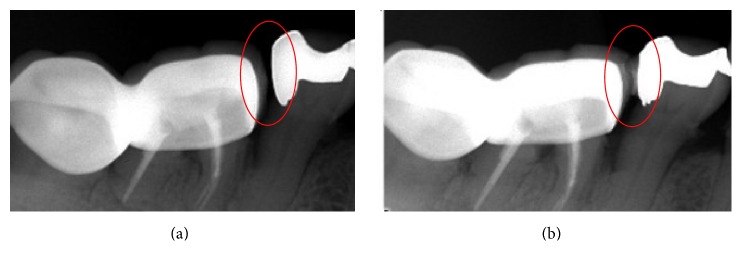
X-ray diagnosis of the interproximal contact area before (a) and after (b) the treatment.
